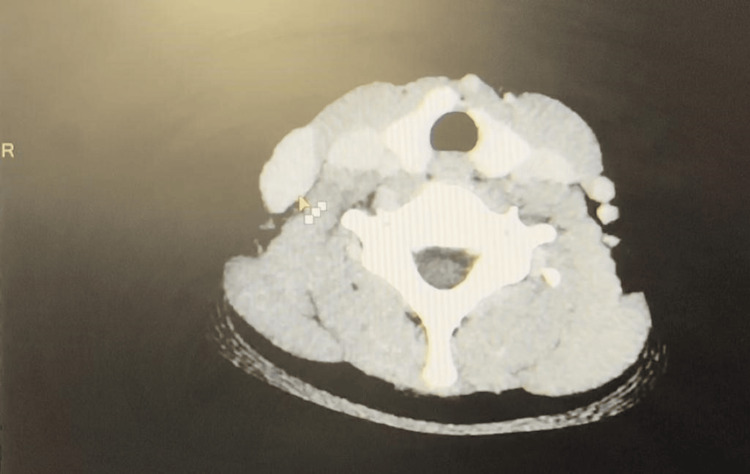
CT image showing slow-flow venous malformation of the right external jugular vein
